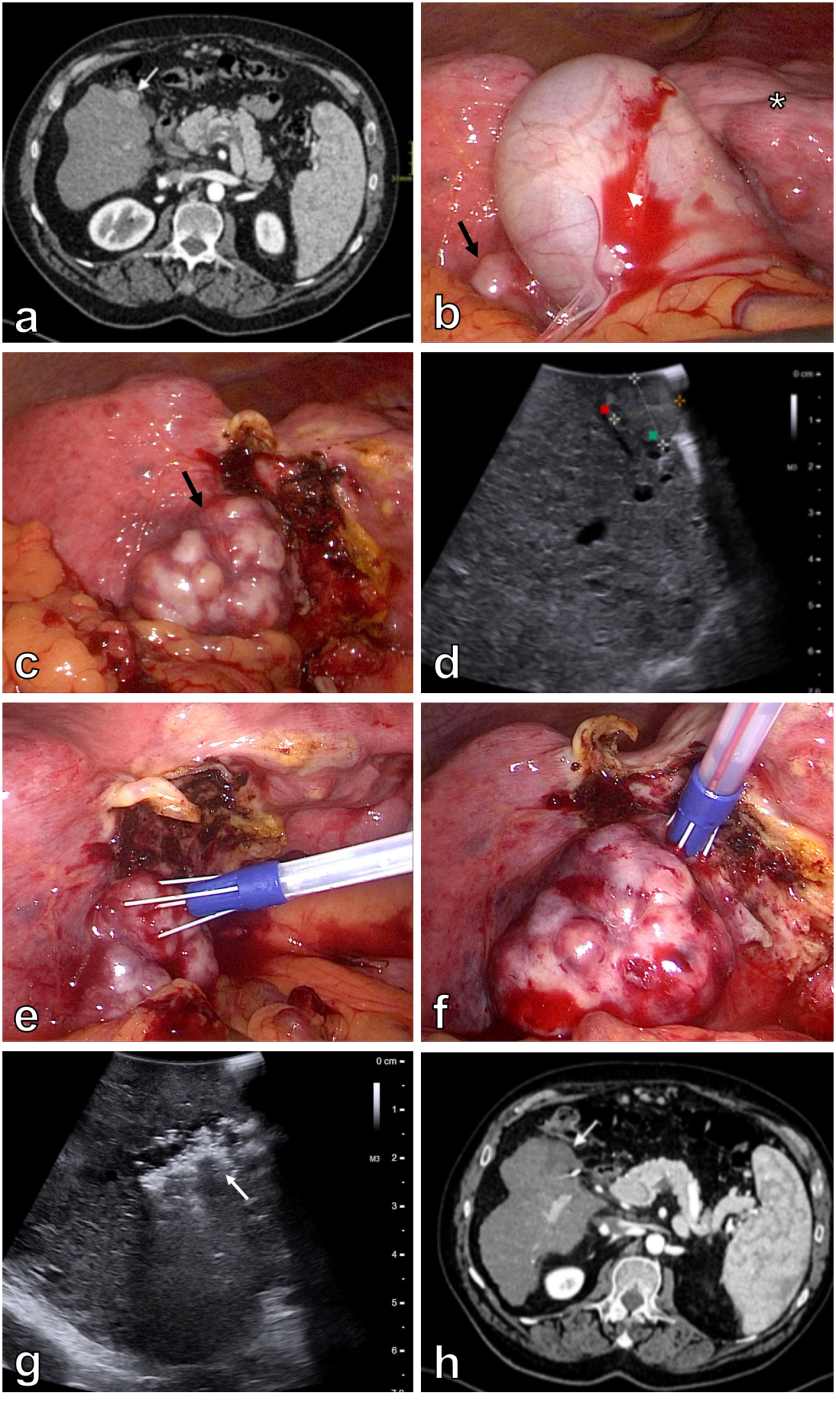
Patient 1. Initial contrast enhanced abdominal CT demonstrates a 12 x 18 mm hypervascular lesion in segment V of the cirrhotic liver (white arrow) (A). Laparoscopy shows macronodular liver cirrhosis (asterisk) with an exulcerated tumor in segment V (black arrow). The majority of the tumor is covered with gallbladder (white arrowhead) (B). Optimal exposure of the tumor (black arrow) is achieved after cholecystectomy (C). Intraoperative US better demonstrates a 22 mm lesion in segment V of the liver (D). Electric pulses are applied through 10° divergent Stinger electrode inserted in the center of the tumor (E) and around the tumor (F). Control intraoperative US immediately after the treatment demonstrates area of avital lesion (white arrow) (G). Contrast enhanced abdominal CT four months after the procedure demonstrates a 35 mm area of ablation necrosis in segment V of the liver (H).
